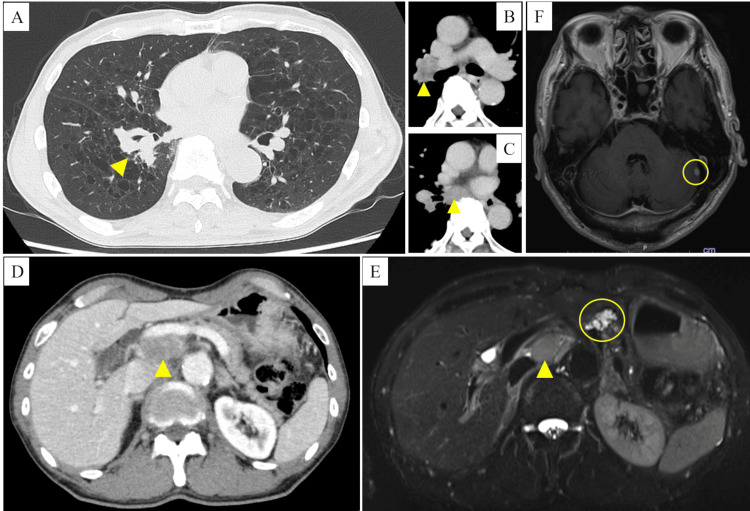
Contrast-enhanced computed tomography, magnetic resonance cholangiopancreatography, and gadolinium-enhanced head magnetic resonance imaging at diagnosis Contrast-enhanced computed tomography at diagnosis showing the tumor in the right lower lobe (yellow arrowhead) (A), right hilar and mediastinal lymphadenopathies (yellow arrowheads) (B, C), and intraabdominal lymphadenopathy (yellow arrowhead) (D). Magnetic resonance cholangiopancreatography showing intraabdominal lymphadenopathy (yellow arrowhead) and pancreatic cysts in pancreatic head (yellow circle) (E). Gadolinium-enhanced head magnetic resonance imaging showing the tumor in the left cerebellum (yellow circle) (F).
